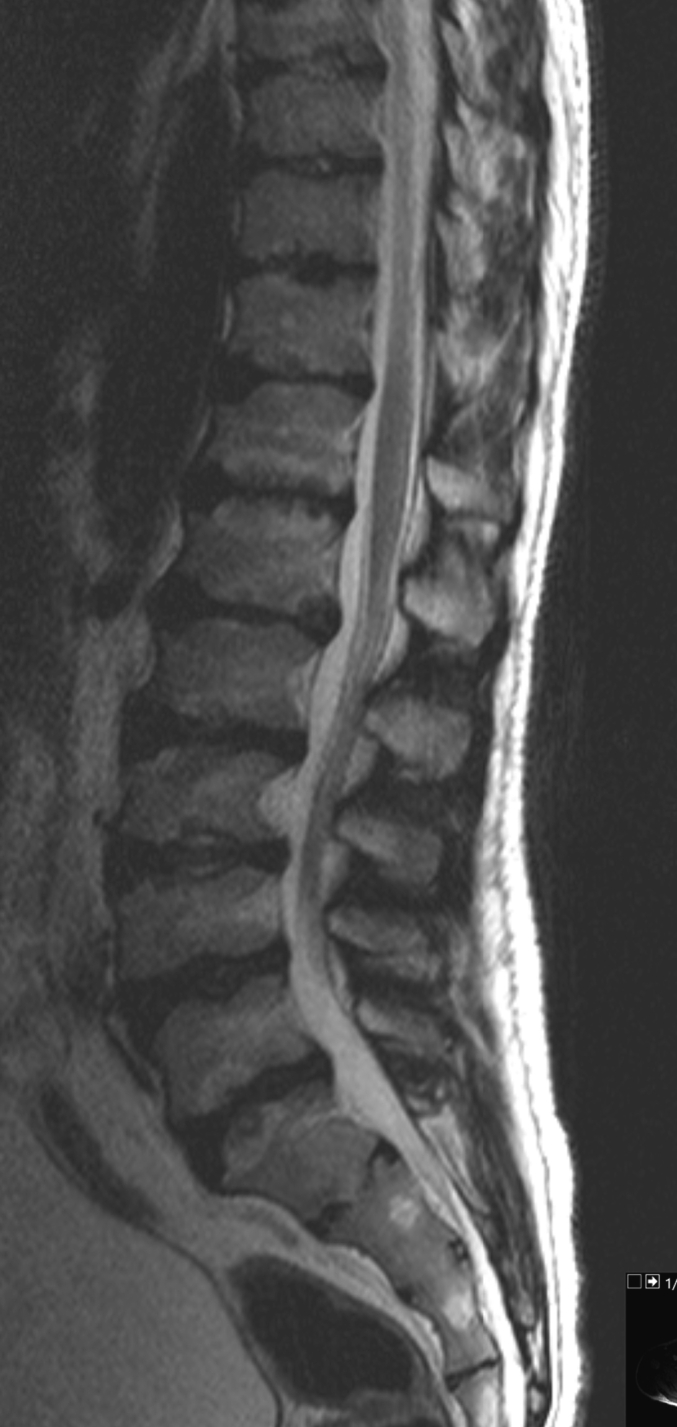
a - MRI spine: dysostosis multiplex in Case 1 (age 20); b- Dysostosis multiplex in lumbar spine in Case 1 (age 20).
